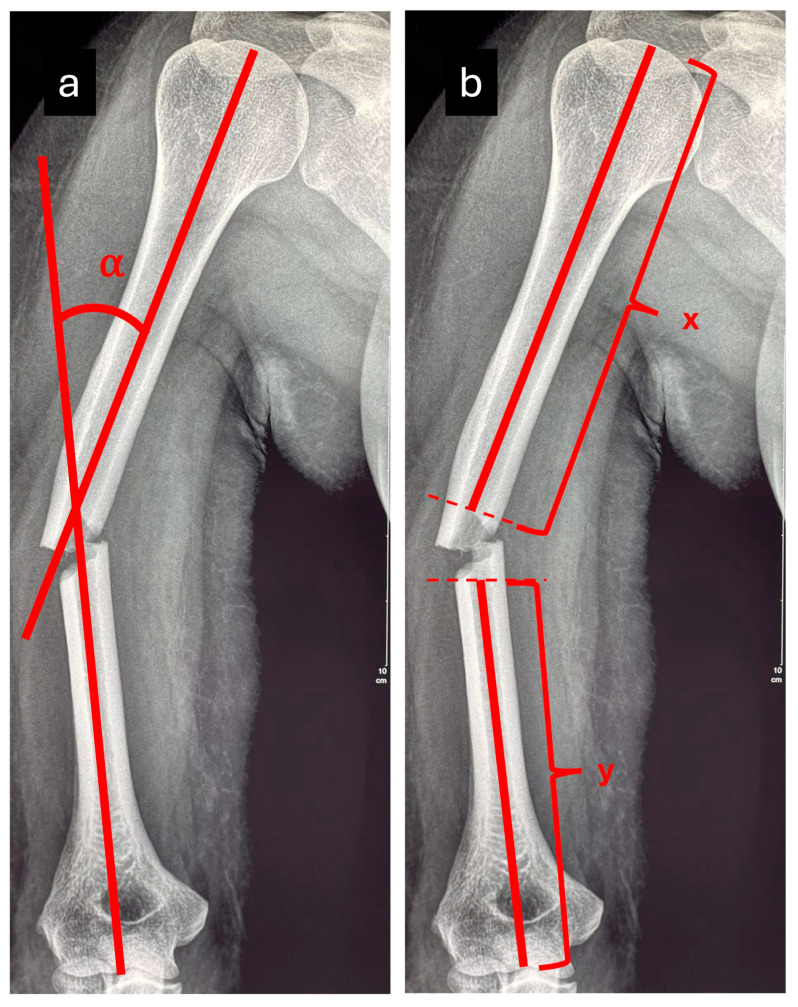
(a) The Cobb angle (α) was measured as the angle between the longitudinal axes of the proximal and distal fracture fragments on the anteroposterior radiograph. (b) The proximal fracture distance (x) was measured from the proximal humeral articular surface to the most proximal extent of the fracture, whereas the distal fracture distance (y) was measured from the distal humeral articular surface to the most distal extent of the fracture.
